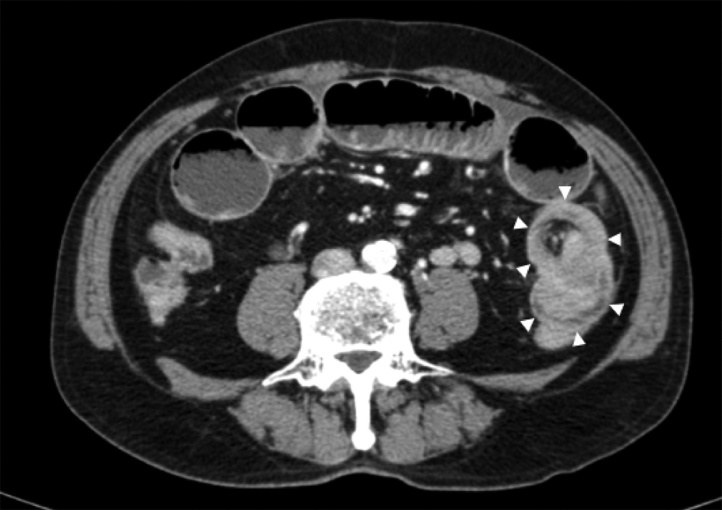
Enhanced abdominal computed tomography detected intussusception in the left lower quadrant with a tumor of 5 cm in diameter in the advanced part (white arrow head).
